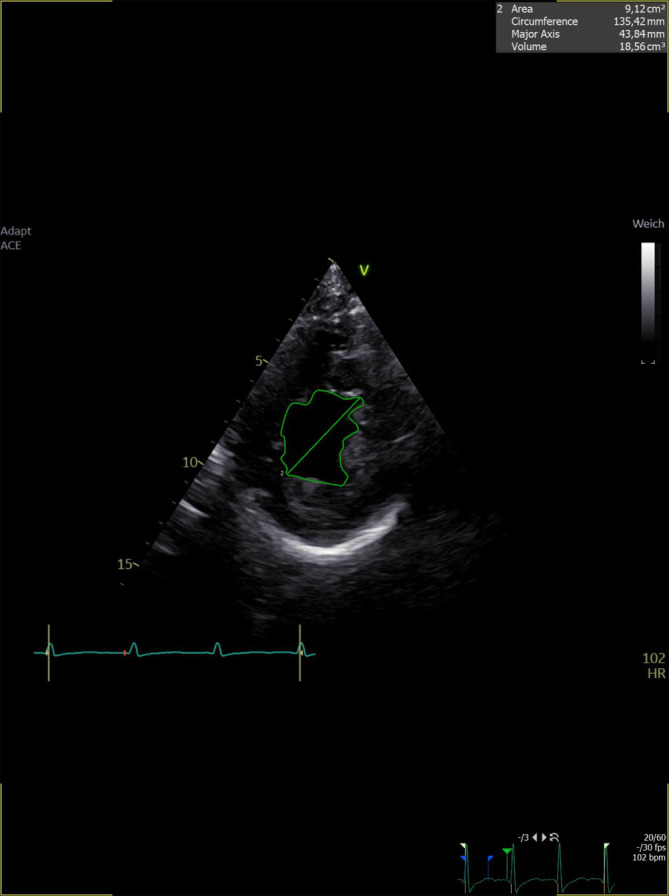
Messung der linksventrikulären enddiastolischen Fläche im parasternalen Kurzachsenschnitt
